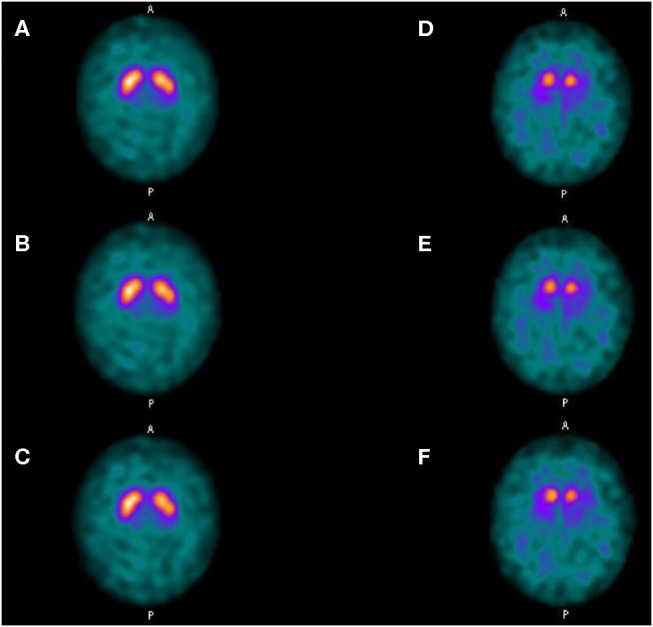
Clinical results. Axial views of a normal patient (A–C) and of a patient suffering from Parkinson disease (D–F). (A,D) SPECT acquisitions with LEHRS collimator without motion. (B,E) SPECT acquisitions with LEHRS collimator with motion (i.e., Swiftscan acquisition). (C,F) SPECT acquisitions with LEHRS collimator with motion, with a 25%-time reduction on each projection.
